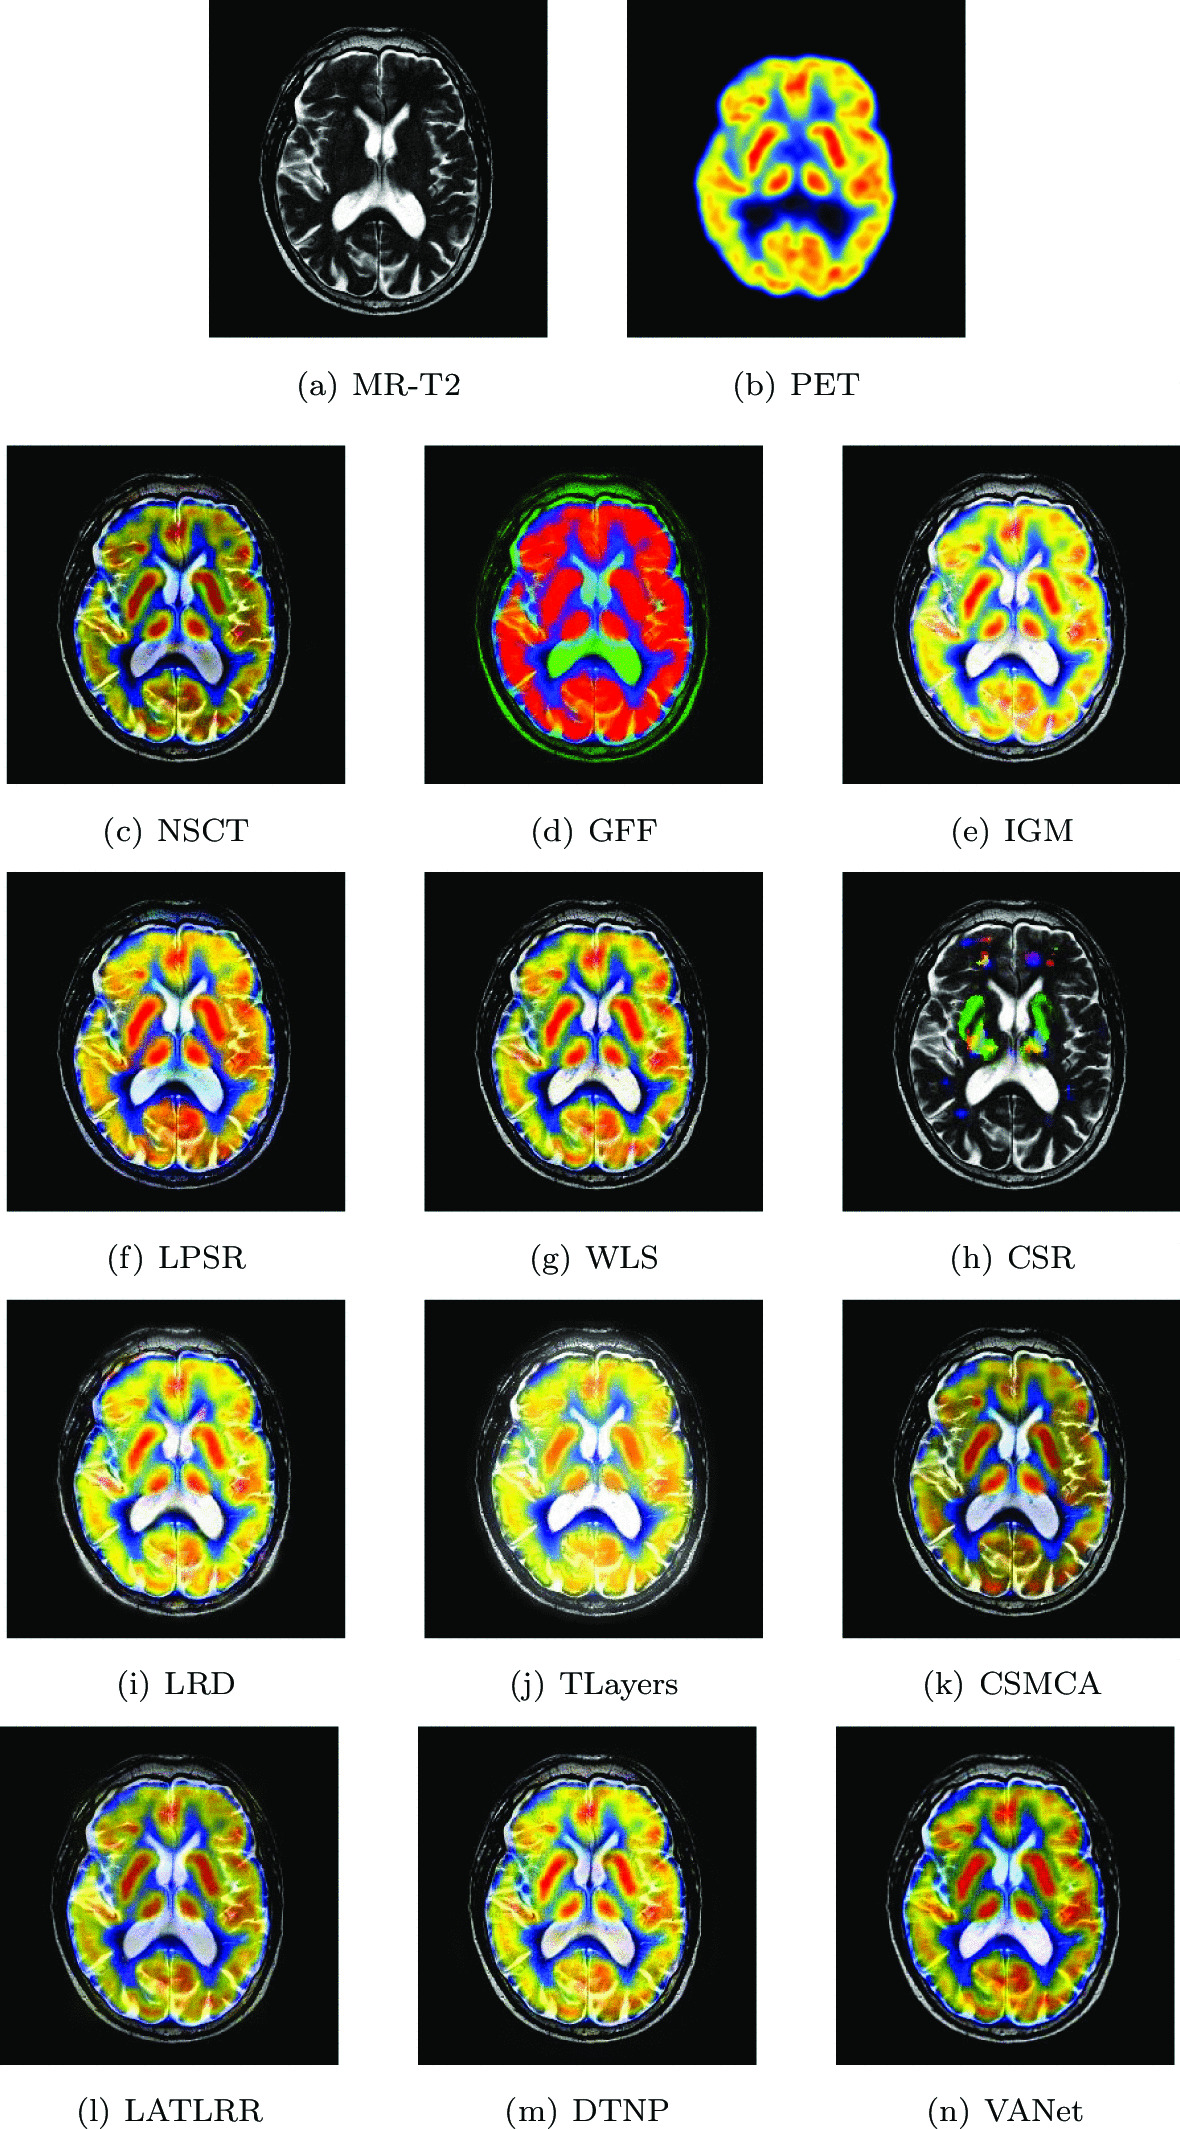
The second set of fused MRI-PET images from 12 methods on mild Alzheimer’s disease
